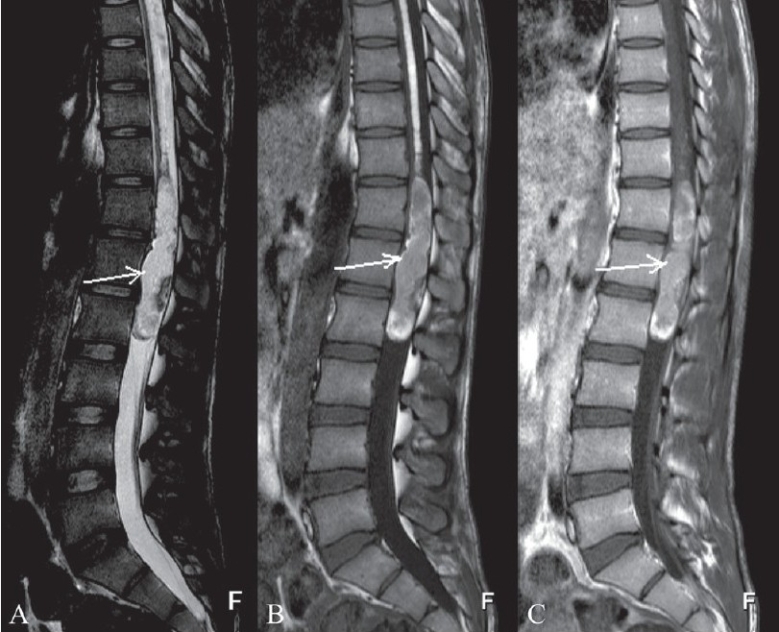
Sagittal T2W (A) and T1W (B) MRI images show a heterogeneous signal intensity mass (arrows) in the conus medullaris region with heterogeneous enhancement in the post-contrast T1W fat-saturation image (C).
